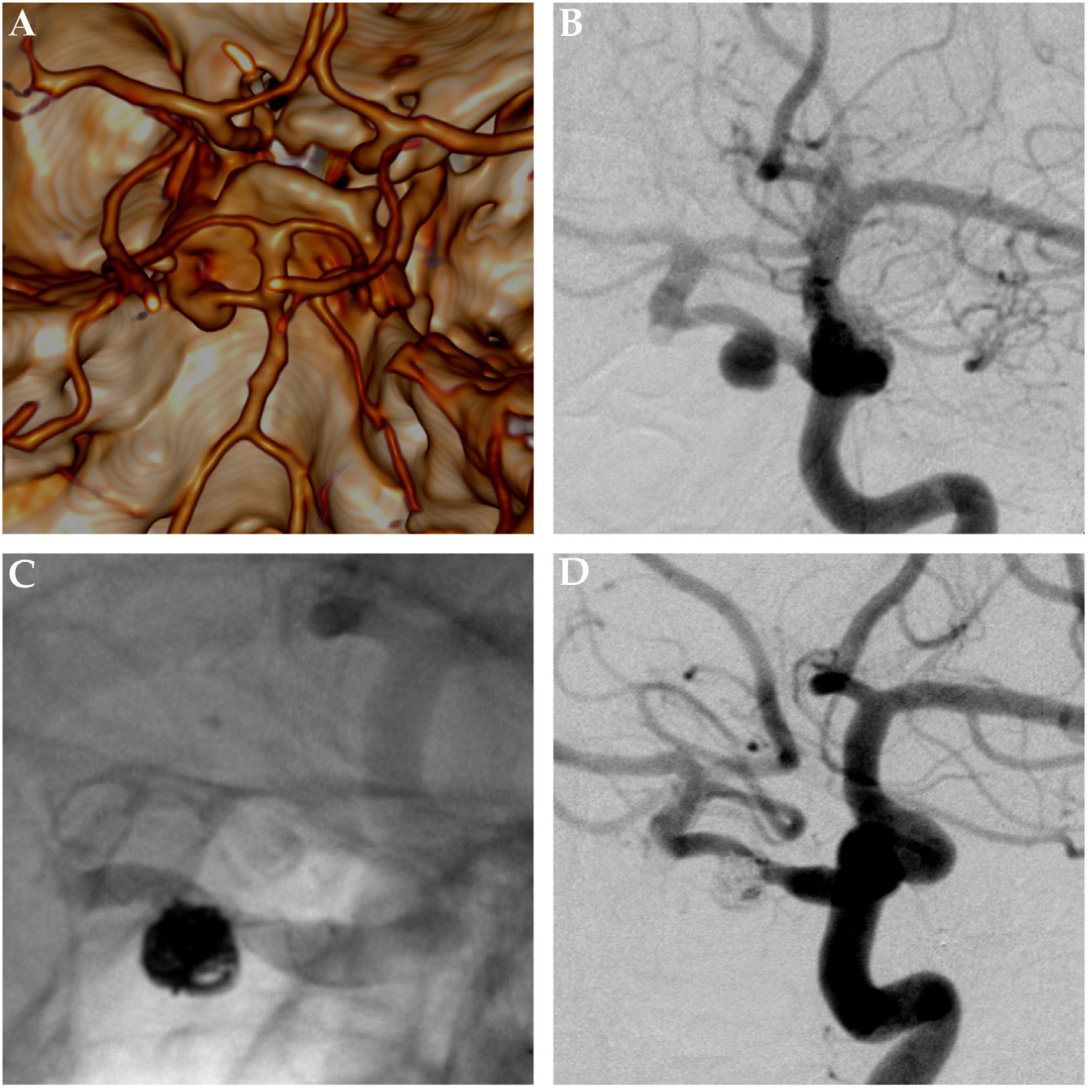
Saccular aneurysm of a primitive trigeminal artery. Panels (A,B) show pretreatment images of a saccular aneurysm of a right-sided primitive trigeminal artery aneurysm. Panel (A) is the reconstructed and volume rendered CT-angiography. Panel (B) gives a posterior–anterior DSA projection. Panel (C) shows the nonsubtracted posttreatment control DSA image, nicely demonstrating the helical radiopaque markers of the low profile self-expandable stent and the radiopaque mass of platin microcoils, sufficiently excluding the aneurysm from the intracranial circulation. Panel (D) shows the corresponding subtracted DSA image. The formerly aneurysm-carrying vessel displays regular endoluminal contrast filling and now reveals signs of periprocedural damage.
